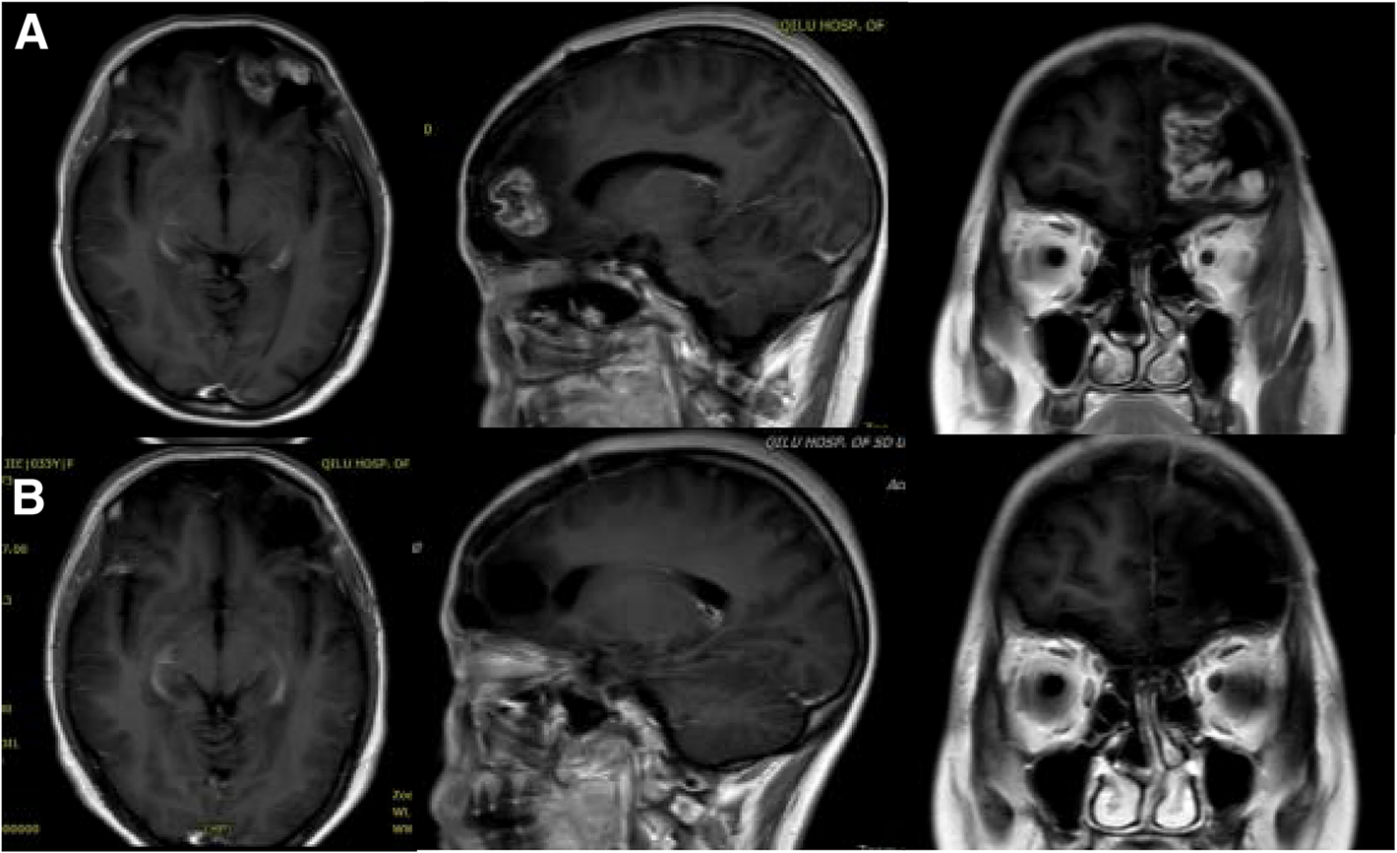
Case 16. Performed on the axial, sagittal, coronal, and enhanced MRI. a Imaging the postoperative recurrence of the left frontal GBM after first operation for 2 years, and the volume was 13.9 cm^3^. b In 72 h, the axial, sagittal, coronal postoperative-enhanced MRI imaging showed no enhanced area residue
